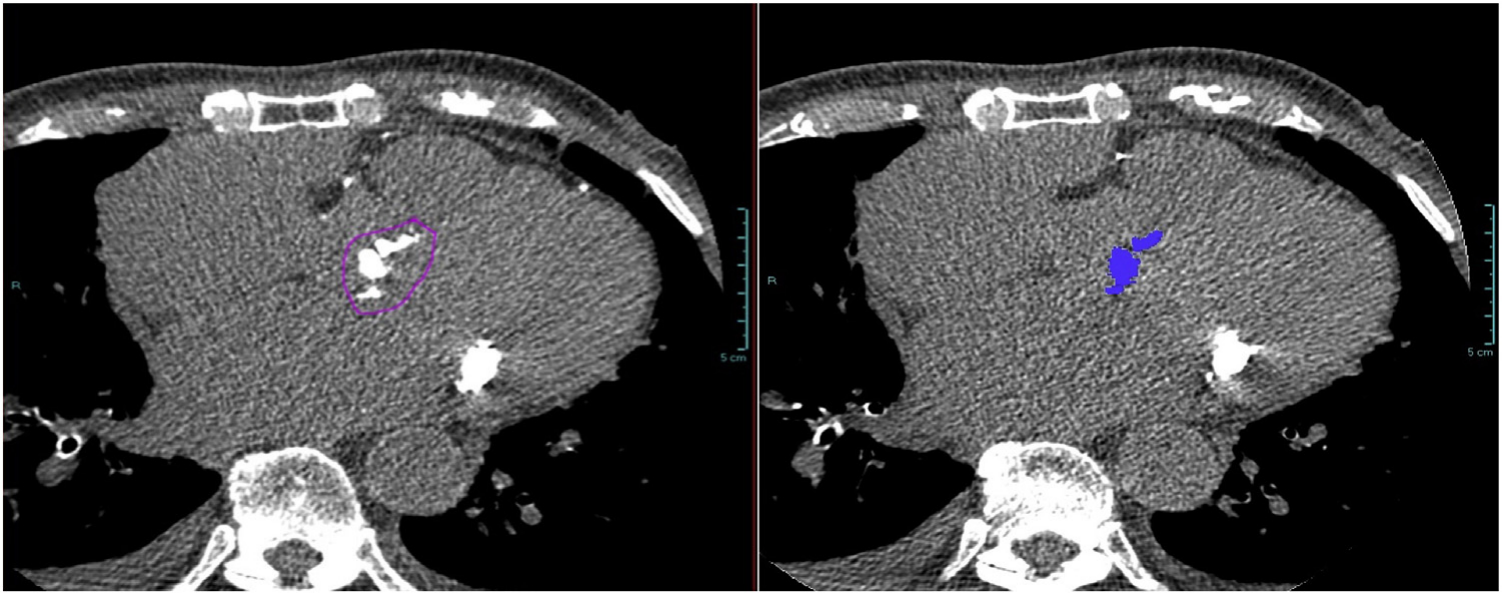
An example 3-mm axial slice from a calcium score sequence of the pre-TAVR CT from a patient in the cohort. CT, computed tomography; TAVR, transcatheter aortic valve replacement.
